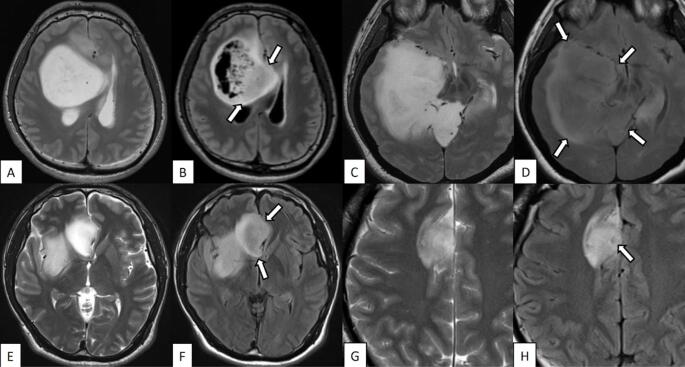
Example cases with positive redefined T2-fluid-attenuated inversion recovery (FLAIR) mismatch sign (T2FM) in astrocytoma, IDH-mutant (AST) and oligodendroglioma, IDH-mutant and 1p/19q-codeleted (ODG). T2-weighted images (A, C, E, G) and FLAIR images (B, D, F, H) are demonstrated in each case (A and B: 40 year-old man, AST, CNS WHO grade 3; C and D: 26 year-old woman, AST, CNS WHO grade 3; E and F: 26 year-old man, AST, CNS WHO grade 2; G and H: 32 year-old man, ODG, CNS WHO grade 2). Redefined T2FM is shown in each case; FLAIR-hypointense signal corresponding to T2-hyperintense signal is observed (arrows)
